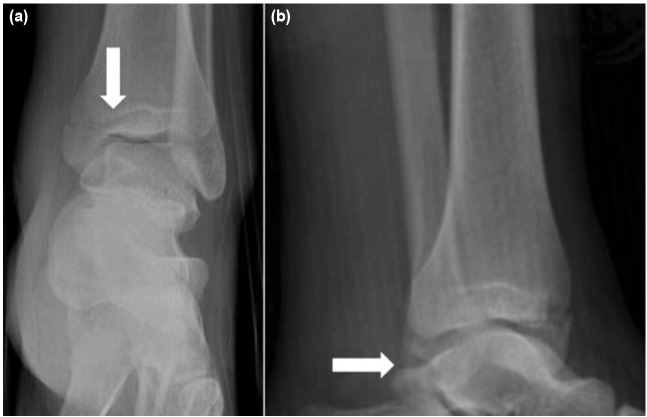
(a) Anteroposterior and (b) Lateral ankle radiographs of the patient on admission showed minimally-displaced fracture of medial malleolus and a suspicious avulsion fracture on posterior part of lateral malleolus.
